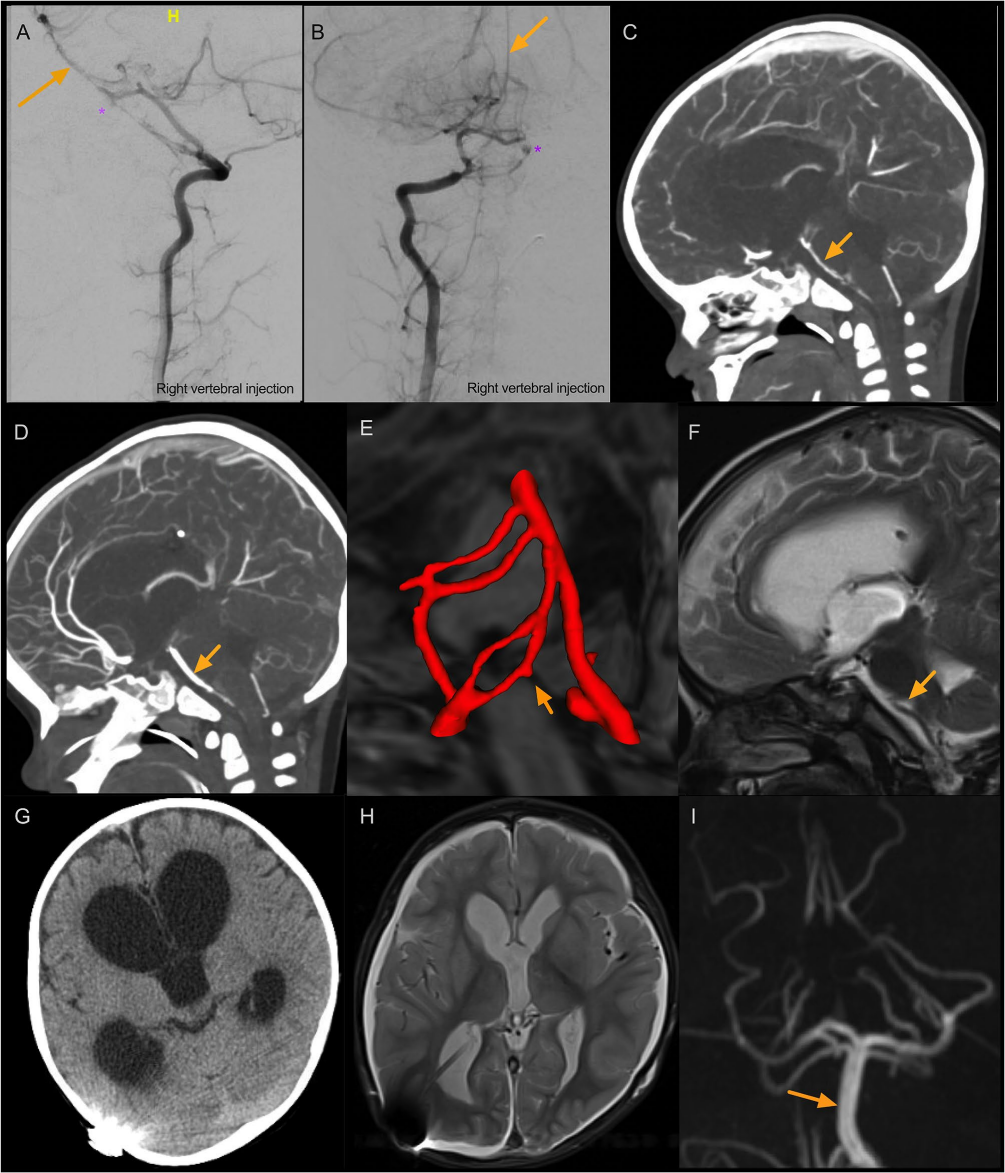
Identification of right intracranial V4 segment vertebral artery aneurysm and vasospasm course. A–B Right vertebral artery injection showing lateral and AP view of aneurysm (*) thought to arise from the left anterior spinal artery and severe vasospasm (orange arrow). C CTA 3 days later showing some more extensive vasospasm now involving the anterior circulation. D CTA 4 days later slowing some resolution of vasospasm. E CTA reconstruction 2 weeks later showing the aneurysm (orange arrow) on the medial arm of the V4 fenestration. F MRA 6 days later showing no aneurysm. G Pre-transfer CT showing maintained ventriculomegary. H–I Interval MRI showing reduction in ventricular calibre, and maintained calibre of posterior circulation vasculature (orange arrow)
